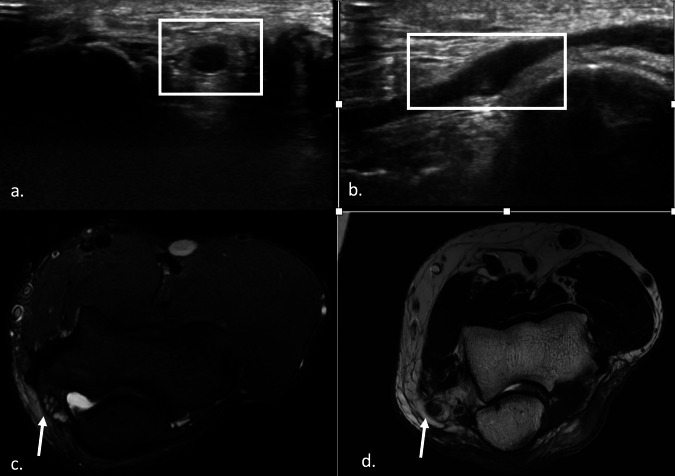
US and MRI features of ulnar compressive neuropathy. Transverse axis US image (a) and longitudinal axis US image (b) of UN at the elbow, showing focal nerve enlargement, loss of the normal honeycomb structure, and decreased echogenicity. Axial fat-suppressed PD (c) and T1-weighted (d) images of UN (arrow) in the cubital canal, showing enlarged UN with hyperintense fascicles (c) and inhomogeneity of the surrounding fat (c, d). In d, the UN is also dislocated anteriorly and medially
